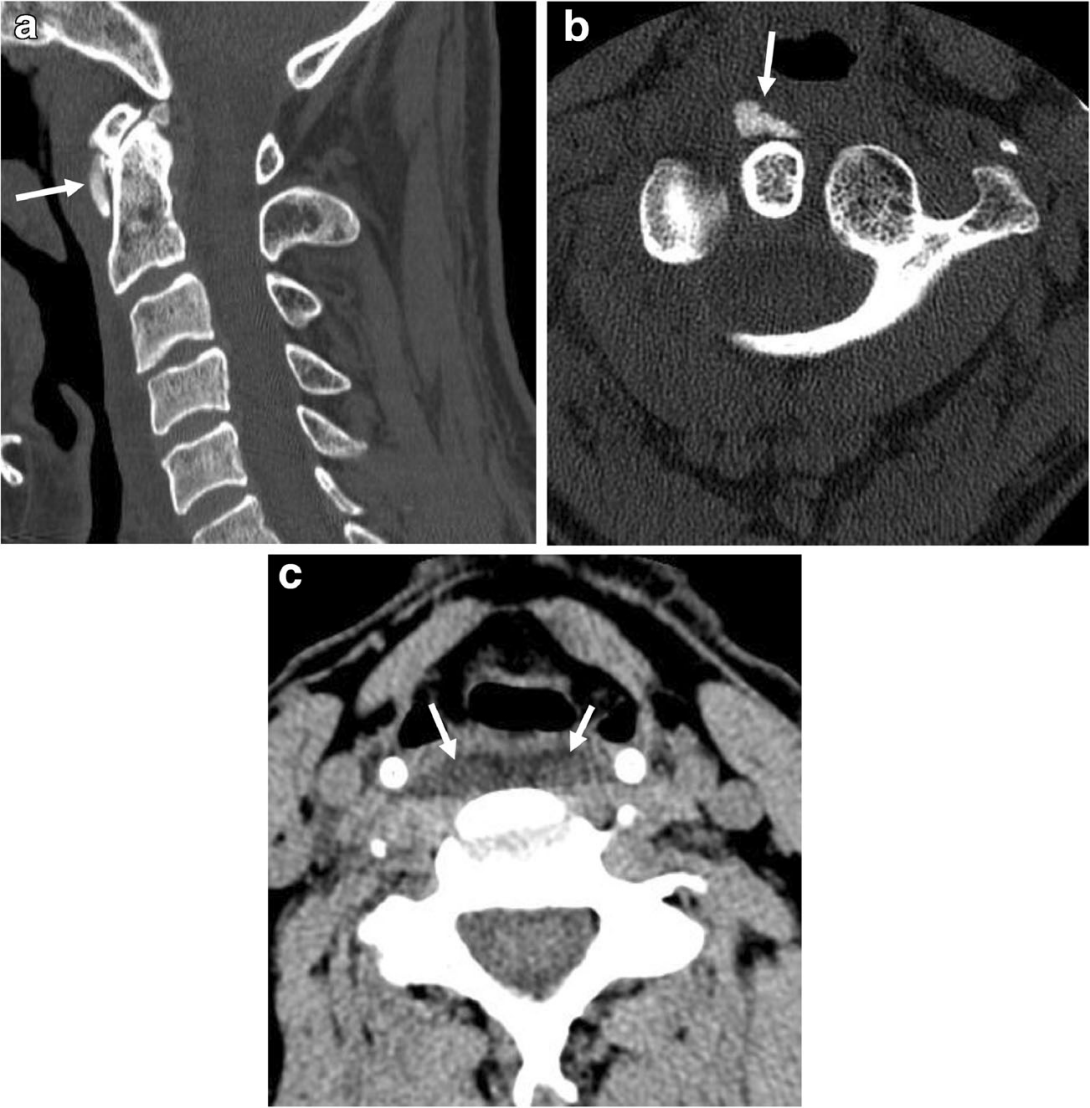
Cervical spine CT examination performed on a 59-year-old male (patient 6) presenting with neck pain and subjective fever without recent trauma. Sagittal (a) and axial (b) images show the characteristic amorphous calcification (*arrows*) of longus colli tendinitis at the upper myotendinous junction of this muscle. Axial image (c) demonstrates symmetric edema (*arrows*) outlining the anterior margin of the longus colli muscles
